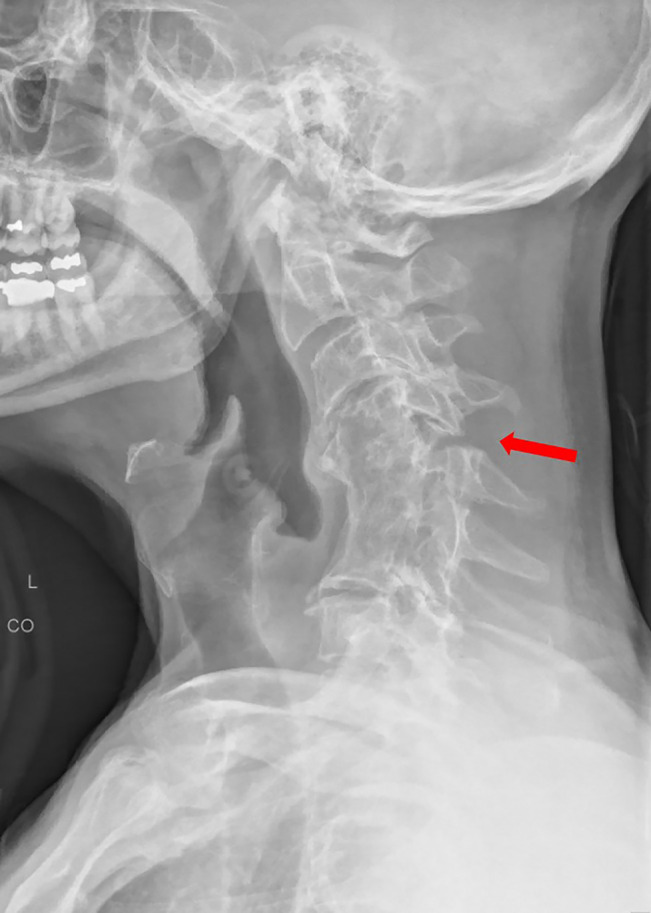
Preoperative standing upright lateral cervical spine radiograph demonstrating
